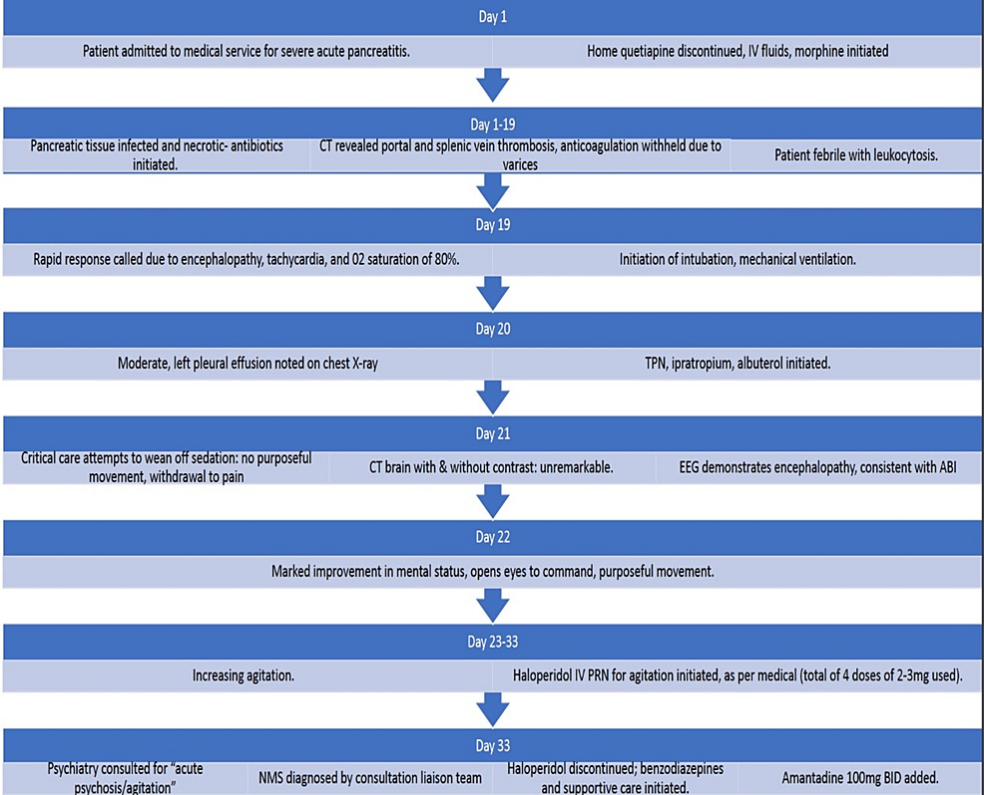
Key points in the patient’s hospital course IV: intravenous, CT: computed tomography, TPN: total parenteral nutrition, EEG: electroencephalogram, ABI: acquired brain injury, PRN: pro re nata, NMS: neuroleptic malignant syndrome, BID: bis in die
